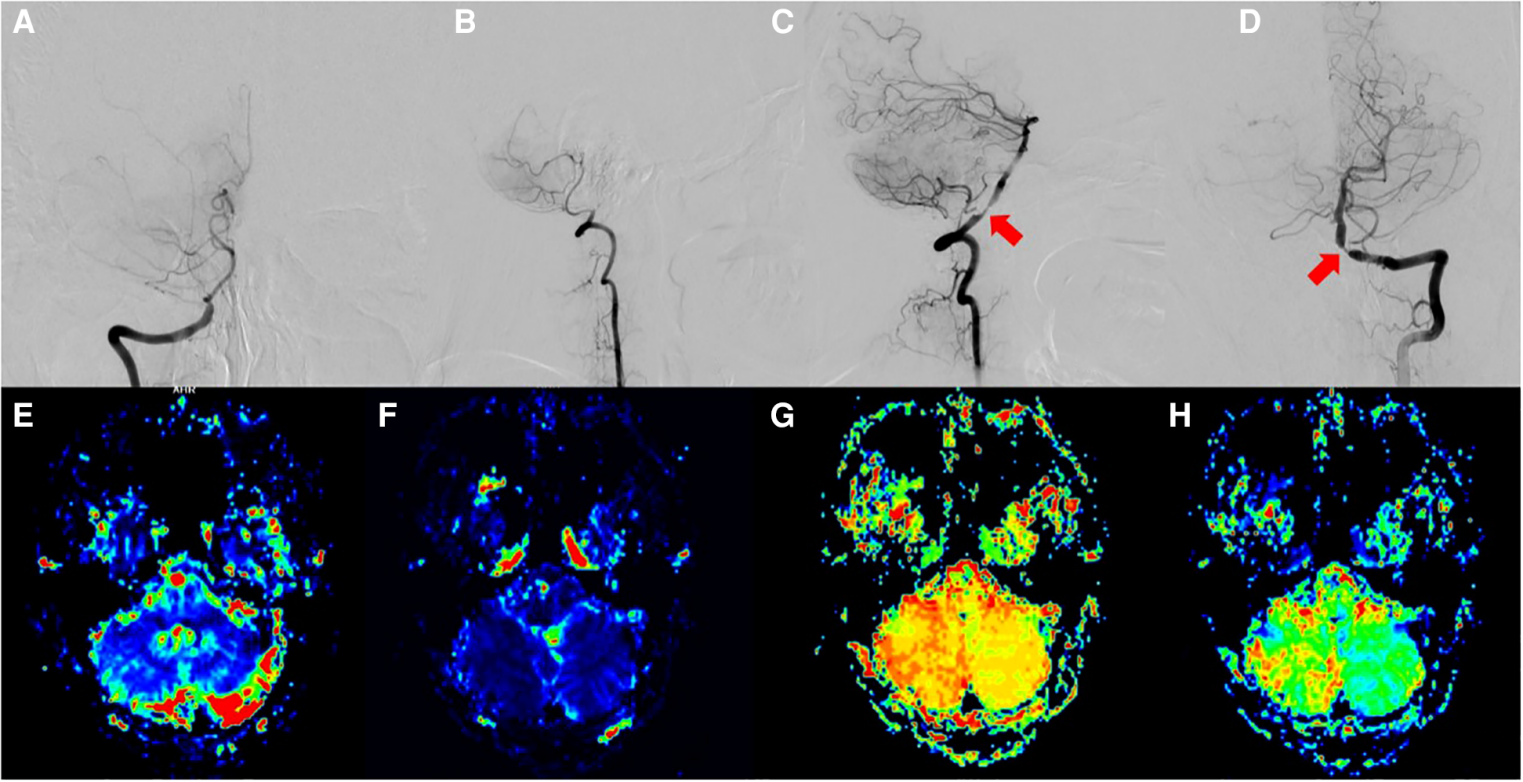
(A,B) Right vertebral artery occlusion; (C,D) Left dominant vertebral artery with stenosis at the opening of the vertebral and PICA arteries; (E–H) MRI, CBV, CBF, TTP, and MTT results indicate bilaterally reduced perfusion and intracranial ischemia.
